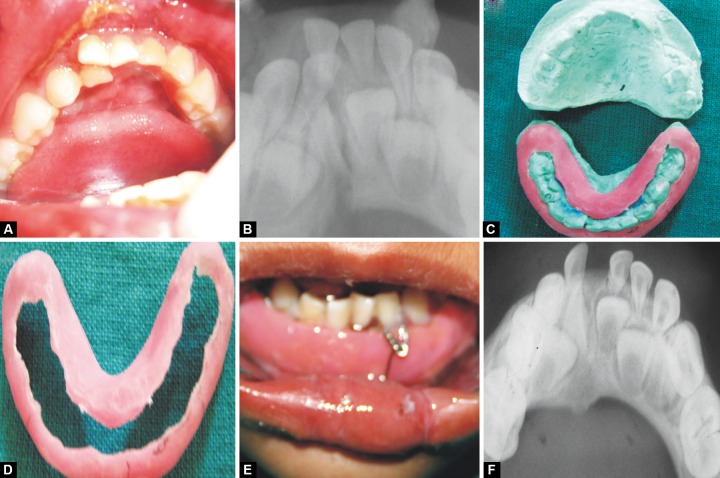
(A) Preoperative photograph, (B) preoperative radiograph, (C) fabrication of MacLennan splint, (D) fabricated MacLennan splint, (E) MacLennan splint with circummandibular wiring and (F) follow-up
